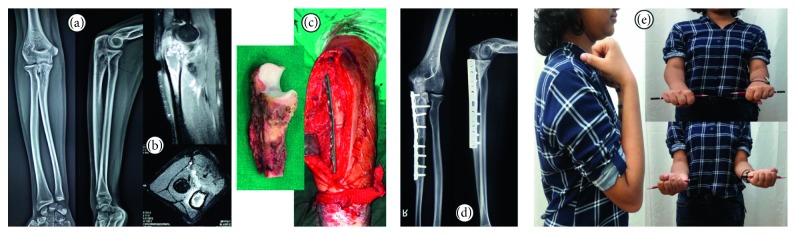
14-year-old female presented with pain in the right elbow, radiographs (a) and MRI (b) confirmed lesion in the olecranon. Biopsy proved Ewing's sarcoma and patient underwent en bloc resection and reconstruction (c) with the extrocorporeal irradiated tumor segment autograft. At latest follow-up, osteotomy junctions have united and no disease/graft- or joint-related complications seen on radiograph (d). Patient has normal range of movement and pronosupination (e).
